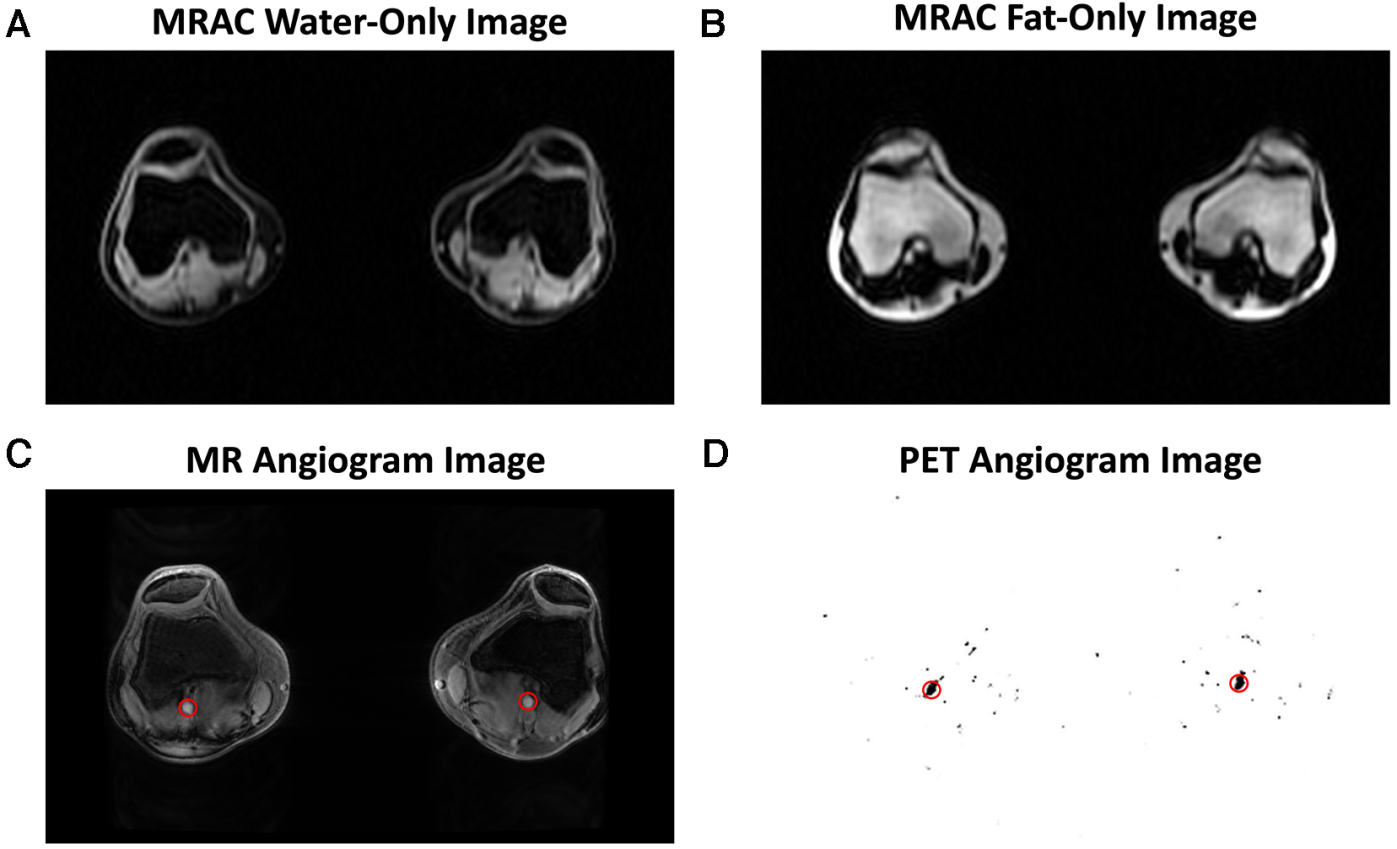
Representative images are shown for the magnetic resonance imaging (MRI) and positron emission tomography (PET) images used in image analysis. MR-based attenuation correction (MRAC) of PET data was performed using a 2-point Dixon fat-water T1-weighted spoiled gradient echo MR sequence, which generated a water-only image (A) and fat-only image (B). An MR angiogram (C) and PET angiogram (D) were used to automatically segment the popliteal arteries (red circles) and derive the arterial input function.
